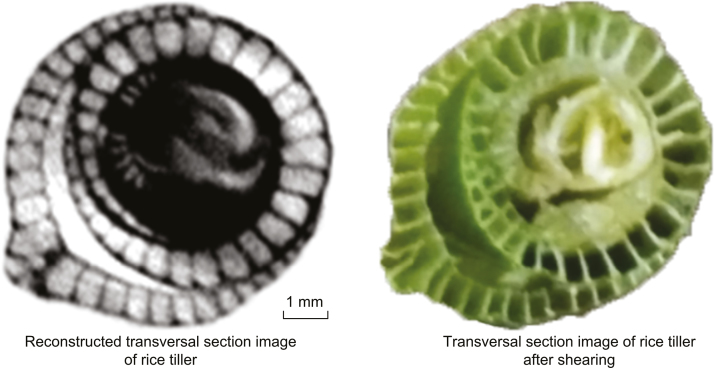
Reconstructed transverse section CT image of a rice tiller at high spatial resolution (30 μm) (left) and transverse section photographic image of the rice tiller after sectioning (right).
